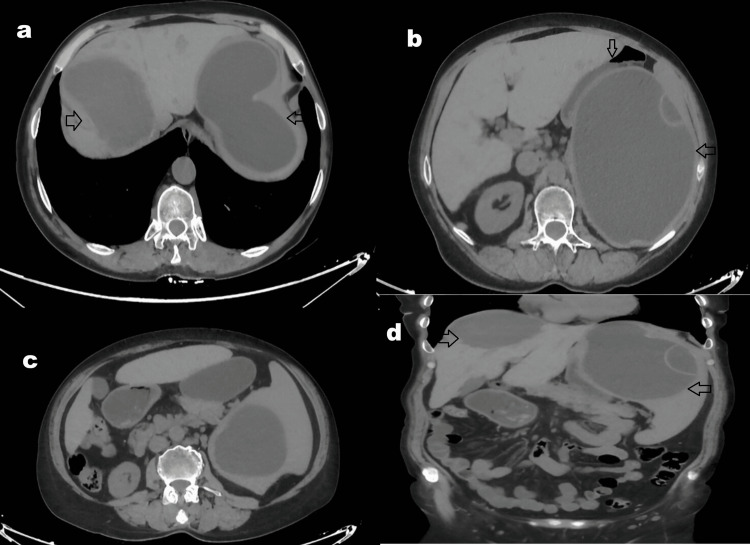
CT images obtained during the conservative follow-up period, approximately three months before surgery. Changes will be added. CT images taken three months before surgery show an isolated large cyst in the right hepatic lobe (a). The cyst in the left hepatic lobe involves both the anterior wall of the stomach (b) and a large portion of the spleen (c, d). The cysts are not fully calcified. Cyst in the right hepatic lobe (arrow >); cyst extending from the left hepatic lobe to the stomach and spleen (arrow <); stomach (arrow ∨).
